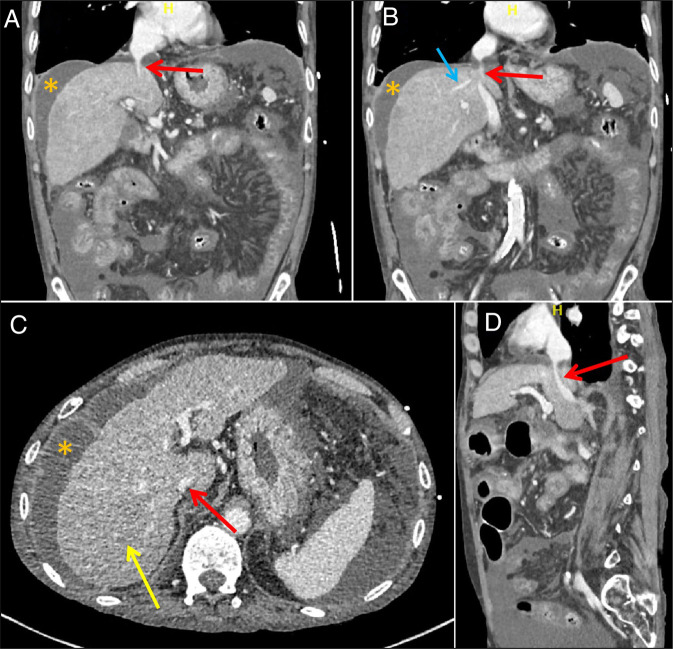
Contrast-enhanced computed tomography images, venous phase. (A and B) Coronal images, revealing IVC stricture (red arrow), ascites (yellow star), and patent hepatic vein (blue arrow). (C) Axial image demonstrating the narrowed IVC (red arrow), ascites (yellow star), and heterogeneous, nodular liver consistent with cirrhosis (yellow arrow). (D) Sagittal projection showing stenotic IVC (red arrow). IVC, inferior vena cava.
